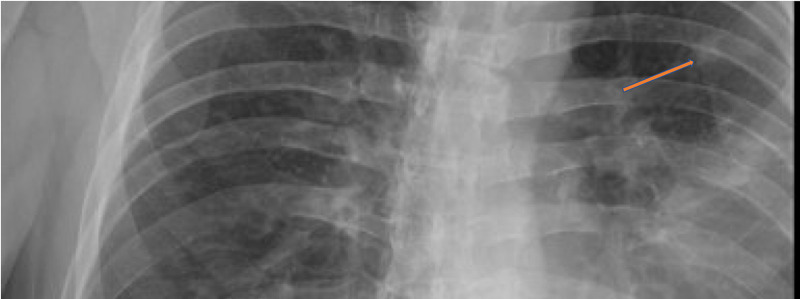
Chest x-ray with left nodular density
